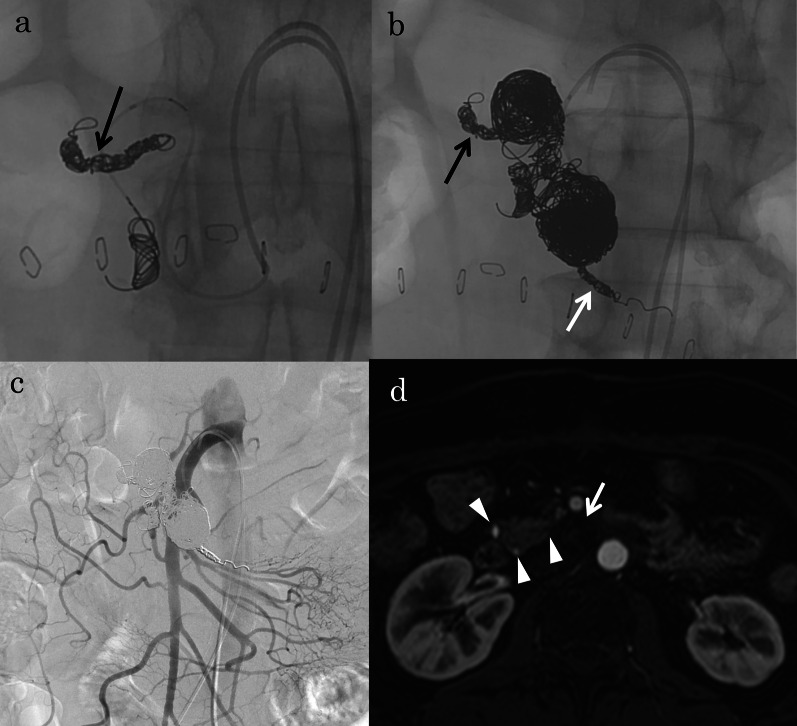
Images from Case 1. a, b Fluoroscopic images during coil embolization showing embolization of the inferior pancreaticoduodenal artery (PDA) combined with packing of the aneurysms. Additional embolization with coils is shown in the branch of the inferior PDA (black arrows) and first jejunal artery (white arrow) arising from the proximal inferior PDA to prevent reperfusion of the PDA aneurysms (PDAAs). c Post-embolization angiogram via the superior mesenteric artery showing complete occlusion of the aneurysms. d Contrast-enhanced magnetic resonance imaging after treatment showing disappearance of the arterial flow in the proximal PDAA (white arrow) and decreased thickness of the pancreatic arcades (white arrowheads)
